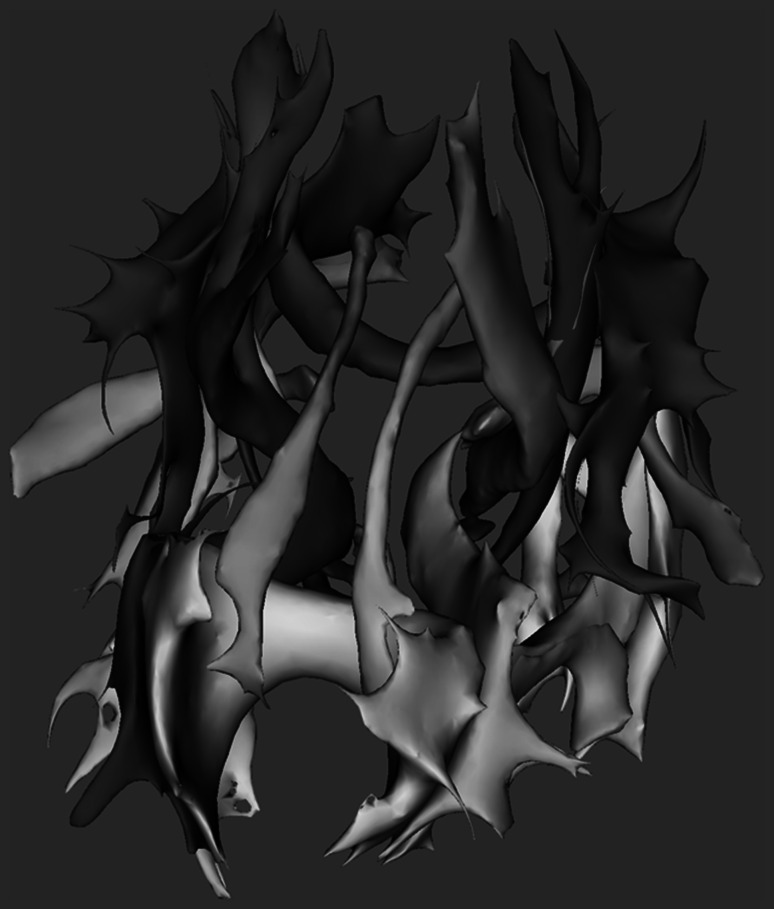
Example of the automatic analysis of diffusion MRI characteristics in 23 white matter structures
